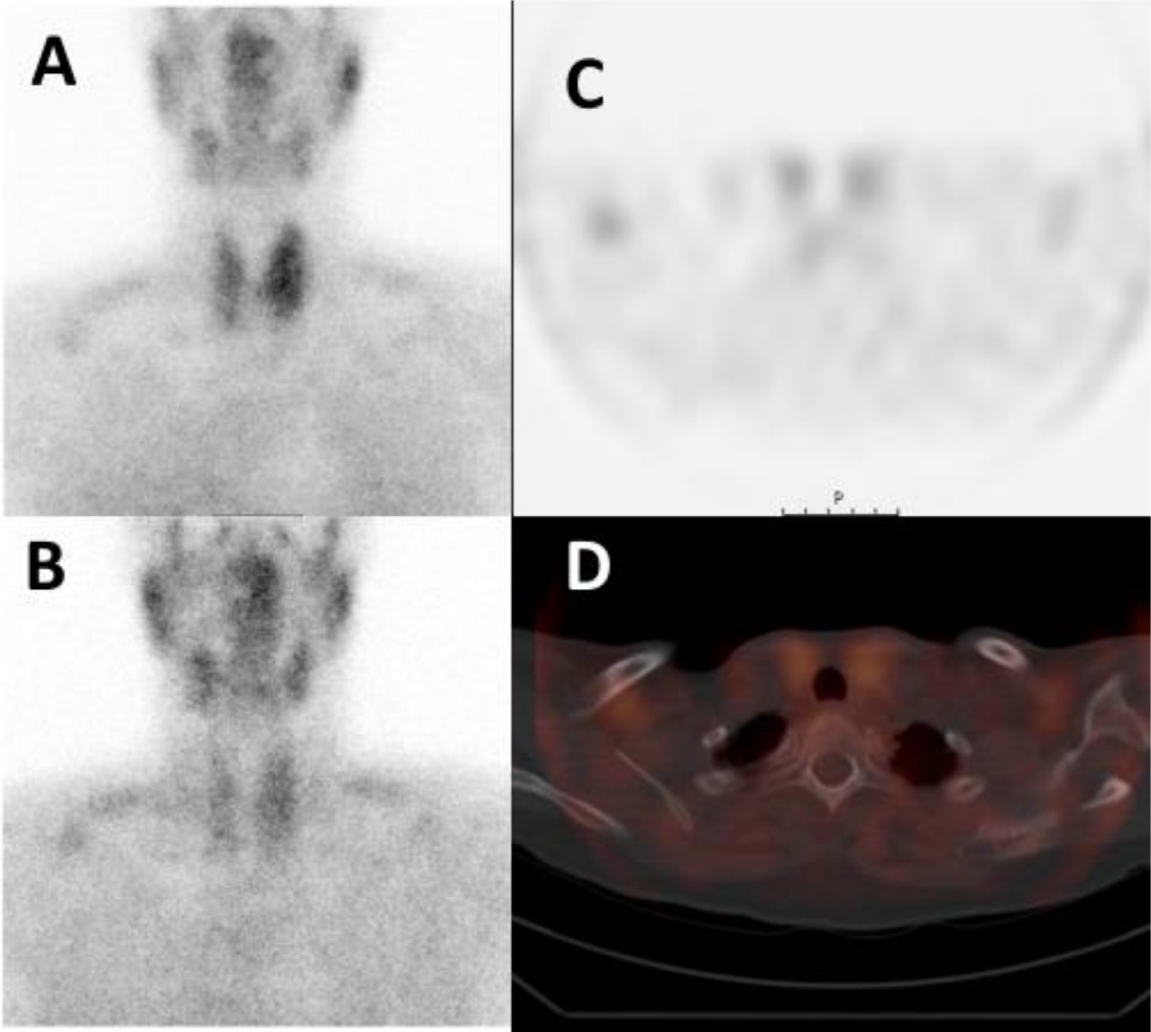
Tc99m-Sestamibi SPECT-CT of patient 2. No increased uptake was seen on the first phase (A) and no retention on the second phase planar images (B). Transversal SPECT (C) and fused SPECT-CT (D) image also show no increased Sestamibi uptake at this lesion.
